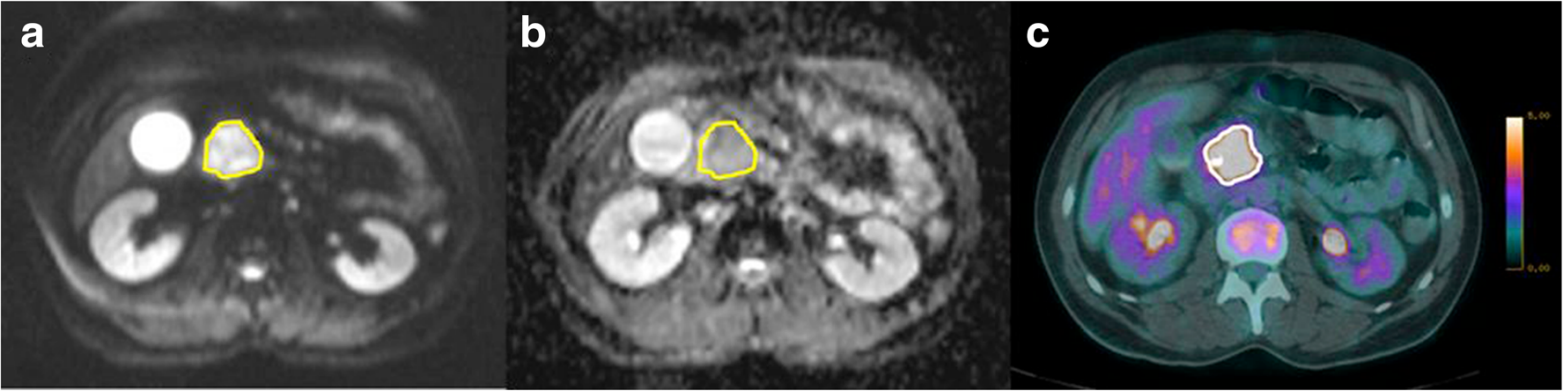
Example of a patient with untreated PDAC. Diffusion-weighted MRI (a, *b*-value: 800 s/mm^2^), ADC map (b), and PET/CT (c) were acquired in a 54-year-old male who underwent cephalic duodenopancreatectomy for a pT2N2M0 pancreatic ductal adenocarcinoma. The ADC_mean_, SUV_mean_, MTV, and MTV/ADC_min_ ratio were 1855 × 10^−6^ mm^2^/s, 5.2 g/mL, 20 mL, and 21 × 10^−3^, respectively
